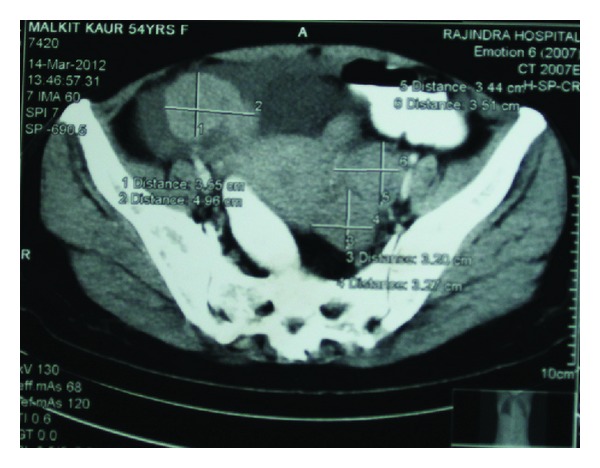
CT showing multiple heterogeneously enhancing masses in the pelvic around the uterus.
